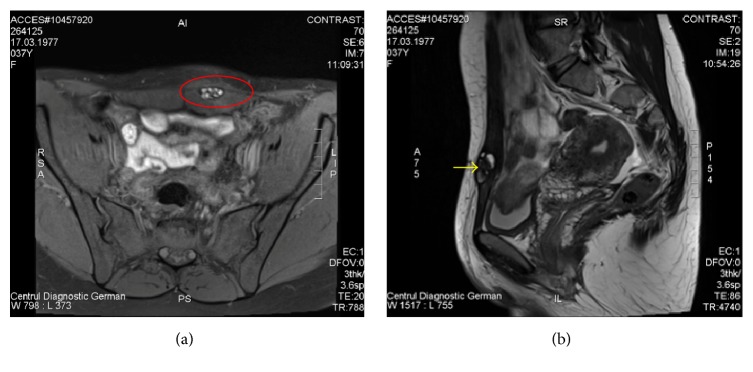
MRI: 21 × 23 × 28 mm heterogeneous mass in the lower left rectus abdominis muscle with focal areas of high and low signal intensity.
